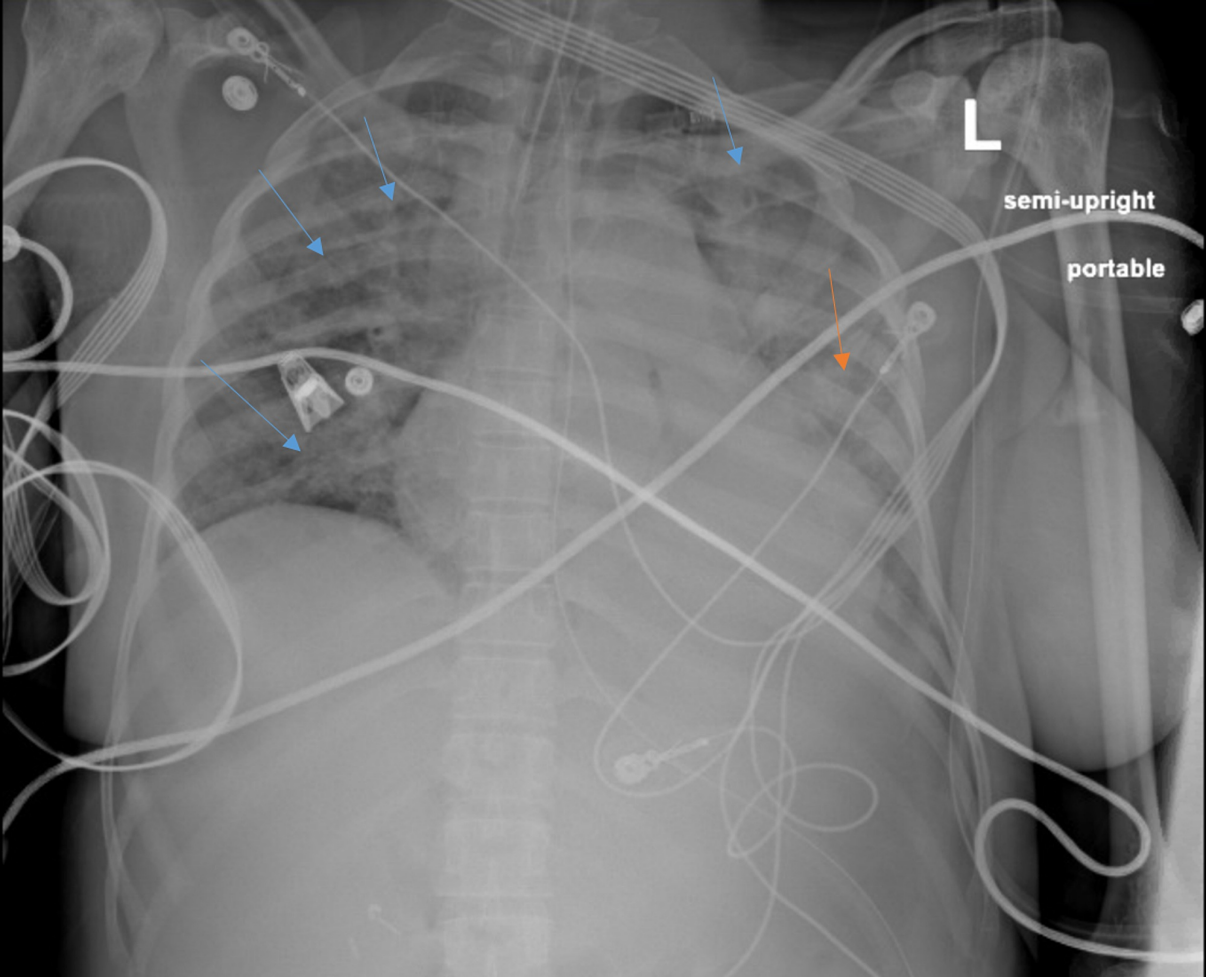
Patient’s chest X-ray after transfer to the ICU post-intubation. New diffuse bilateral infiltrates are noted (blue arrows), new consolidative changes in the left lower lobe are noted as well (orange arrow).
